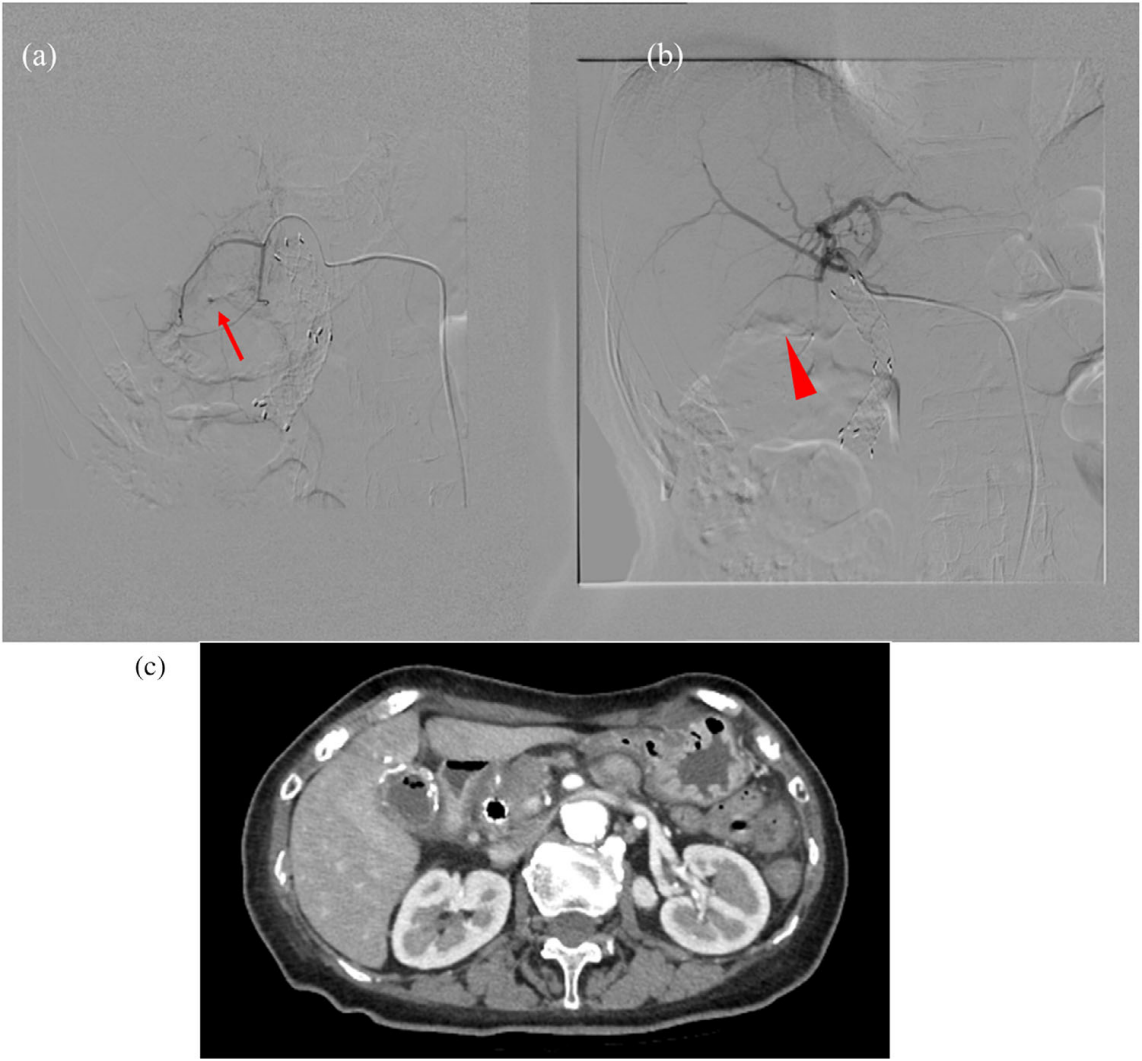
(a) Cystic artery pseudoaneurysm on angiography (arrow). (b) Blood flow to cystic artery pseudoaneurysm disappeared after transcatheter arterial embolization (arrowhead). (c) Contrast‐enhanced computed tomography after transcatheter arterial embolization confirmed the absence of blood flow to the deep cystic artery.
